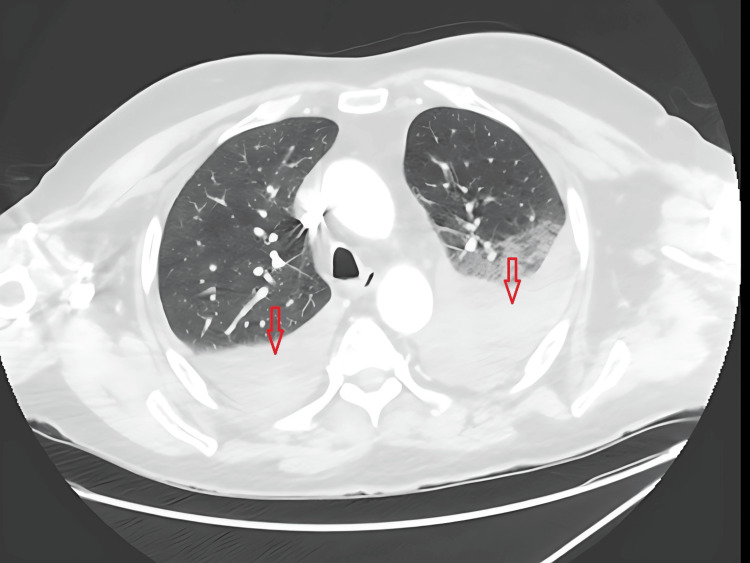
CT scan of chest showed bilateral pleural effusion
